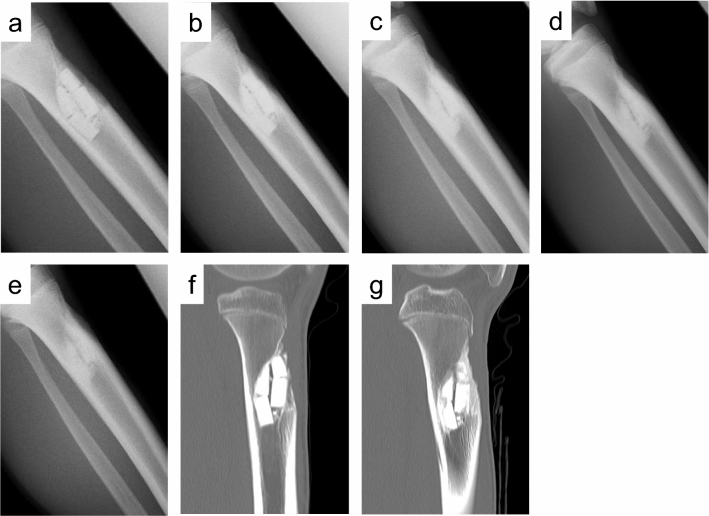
The degrees of Affinos filling the defects were classified into five stages with plain radiographs and computed tomography (CT) images in a 13-year-old boy with tibial fibrous dysplasia. (a) Radiograph at 1 month after surgery: the values of resorption and trabeculation were 0% and 0%, respectively. (b) Radiograph at 3 months after surgery: the values of resorption and trabeculation were 25% and 50%, respectively. (c) Radiograph at 6 months after surgery: the values of resorption and trabeculation were 75% and 75%, respectively. (d) Radiograph at 9 months after surgery: the values of resorption and trabeculation were 75% and 100%, respectively. (e) Radiograph at 12 months after surgery: the values of resorption and trabeculation were 100% and 100%, respectively. (f) Sagittal view of tibial CT at 3 months after surgery: the values of resorption and trabeculation were 25% and 50%, respectively. (g) Sagittal view of tibial CT at 9 months after surgery: the values of resorption and trabeculation were 50% and 75%, respectively.
